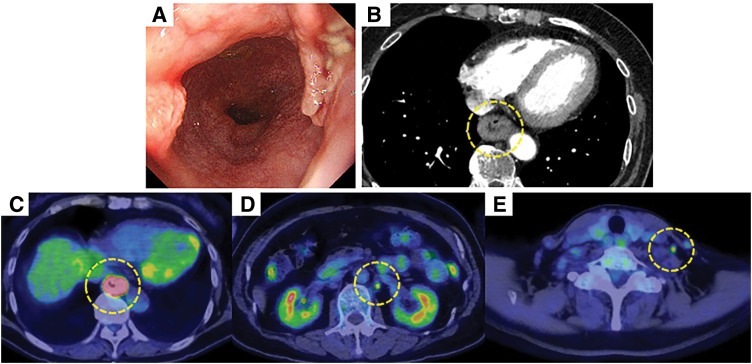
Imaging findings at initial diagnosis. (A) Upper gastrointestinal endoscopy reveals a type 2 lesion centered on the posterior wall of the midthoracic esophagus. (B) Contrast-enhanced CT demonstrates eccentric, near-circumferential wall thickening of the midthoracic esophagus (yellow circle). (C) PET-CT shows increased FDG uptake in the midthoracic esophagus (yellow circle). (D) Abnormal FDG accumulation suggesting lymph node metastasis is observed in the left supraclavicular fossa (yellow circle). (E) Abnormal FDG uptake suggesting lymph node metastasis is noted in the left para-aortic region (yellow circle). FDG, ^18^F-fluorodeoxyglucose
